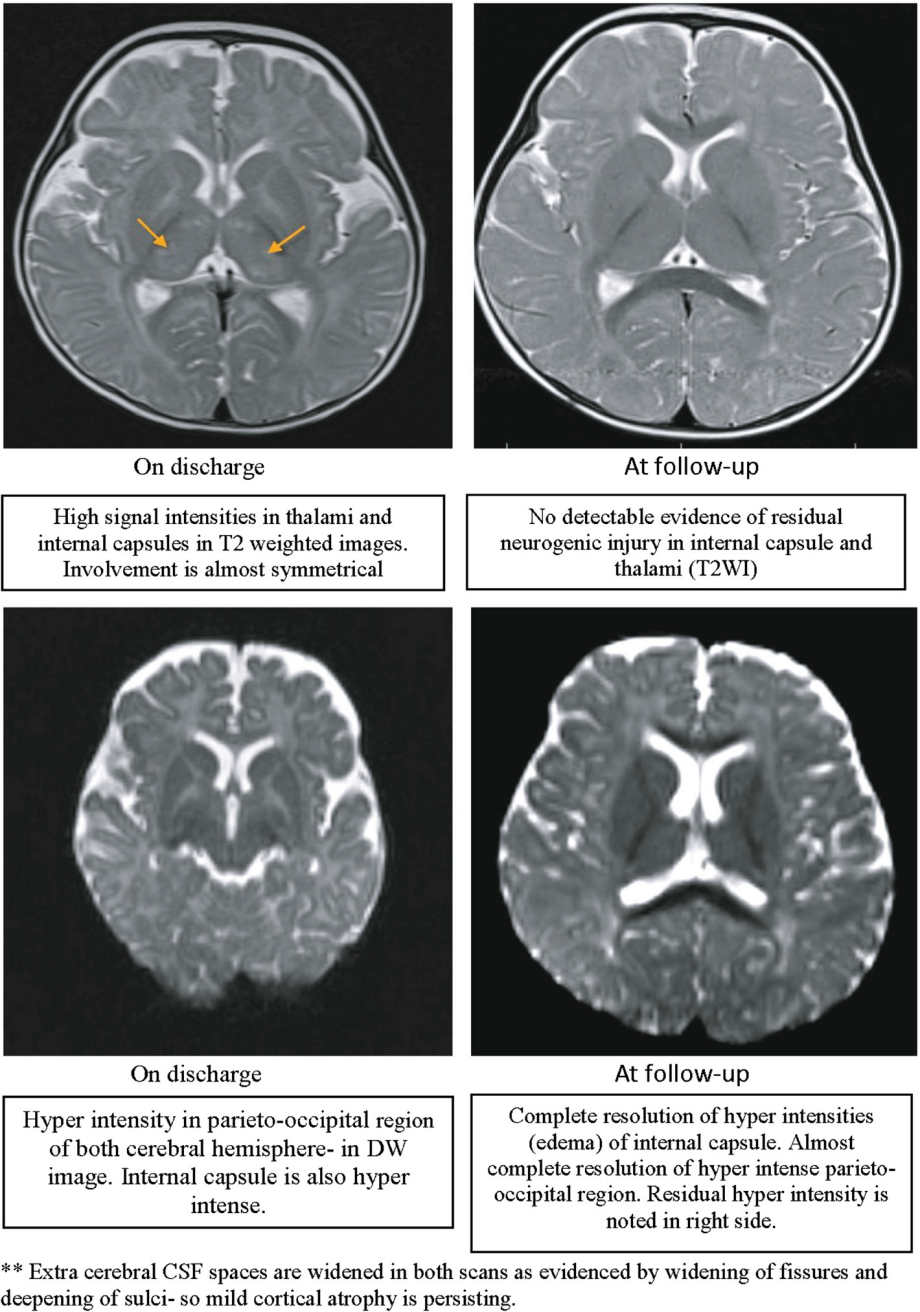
Magnetic resonance imaging scans of the patient’s brain. Images show the acute changes due to hypernatremia at hospital discharge and 3-month follow-up.
